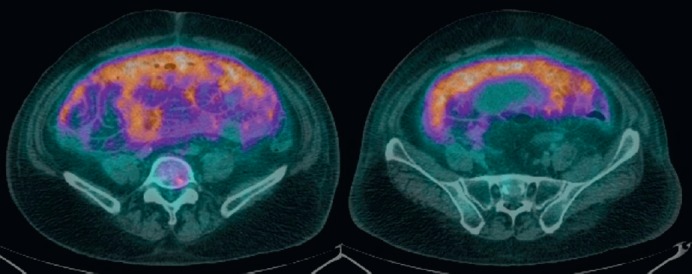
PET/CT scan showing diffuse uptake over peritoneal space (SUVmax from 5.6 to 6.4).
